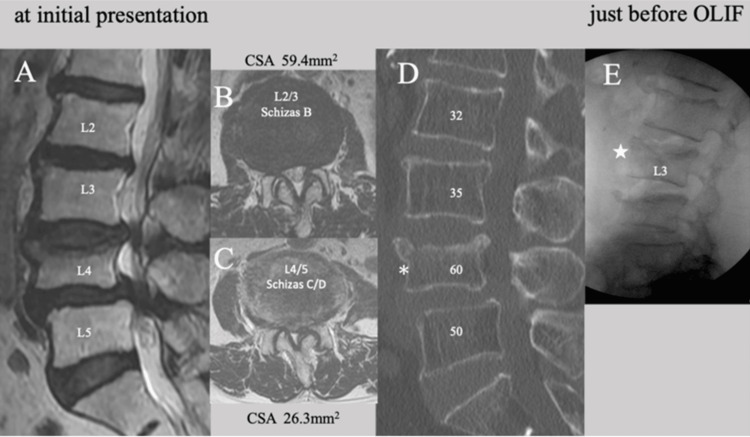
Preoperative MRI and CT images of Case 1 MRI and CT images of an 81-year-old woman (Case 1) showing (A, B) moderate (Schizas B at L2/3) and (A, C) severe canal stenosis (Schizas C/D at L3/4 and L4/5) with an old L4 vertebral fracture (D, asterisk) and degenerative spondylolisthesis, and (D) very low L2-L4 CT-Hounsfield unit values. A lateral lumbar X-ray (E) taken just before OLIF showing a newly onset L3 vertebral fracture (star). OLIF: oblique lumbar interbody fusion, CSA: cross-sectional spinal canal area
